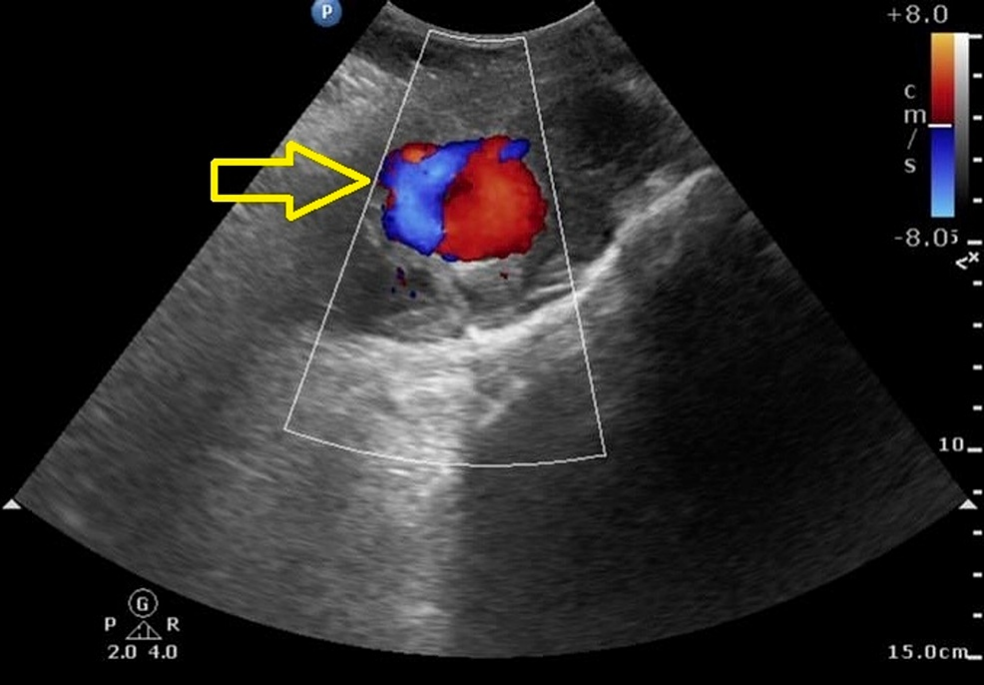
Duplex ultrasound image showing pseudoaneurysm arising from its parent left IEA before thrombin injection.
